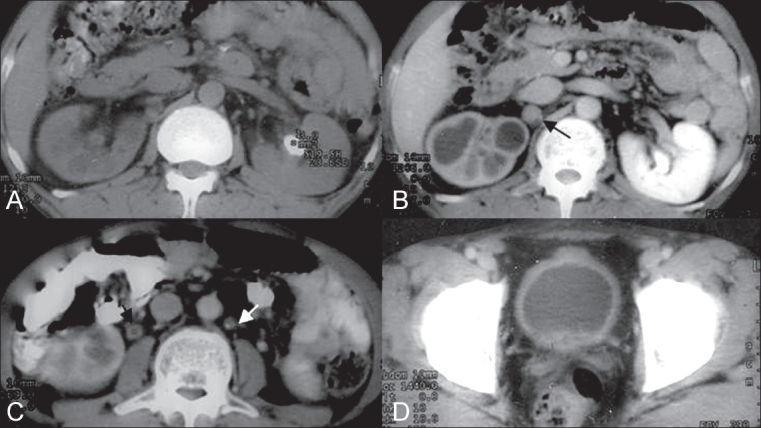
Noncontrast CT image (A) at the level of kidney shows a left renal calculus. A contrast-enhanced CT image (B) shows a hydronephrotic right kidney with mild parenchymal atrophy and a poor nephrogram, along with an enhancing retrocaval lymph node (black arrow). A contrast-enhanced CT image through the lower renal poles shows right ureteritis, with marked wall thickening (black arrow). A partially calcified retroperitoneal lymph node (white arrow) is also seen. A contrast-enhanced CT image at the level of the urinary bladder shows a thickened, enhancing bladder wall, suggestive of cystitis
